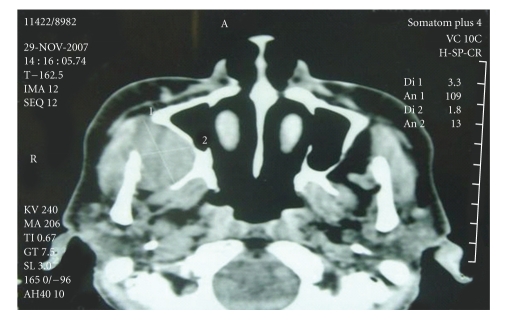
CT scan showing a well limited solid tumor developing from the right infratemporal space indenting upon the posterolateral wall of the right maxillary sinus.
